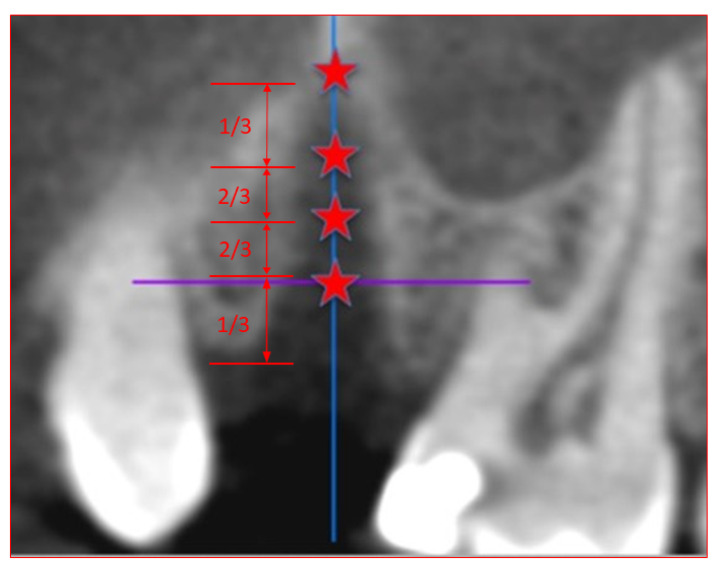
Definition of the measuring points in the cone beam computed tomography scan. The measuring points were located at the junction of coronal third and lower two thirds in the middle and at the junction of apical third and the upper two thirds of the alveoli. Measuring points were arranged on an axis in the center of the alveoli.
